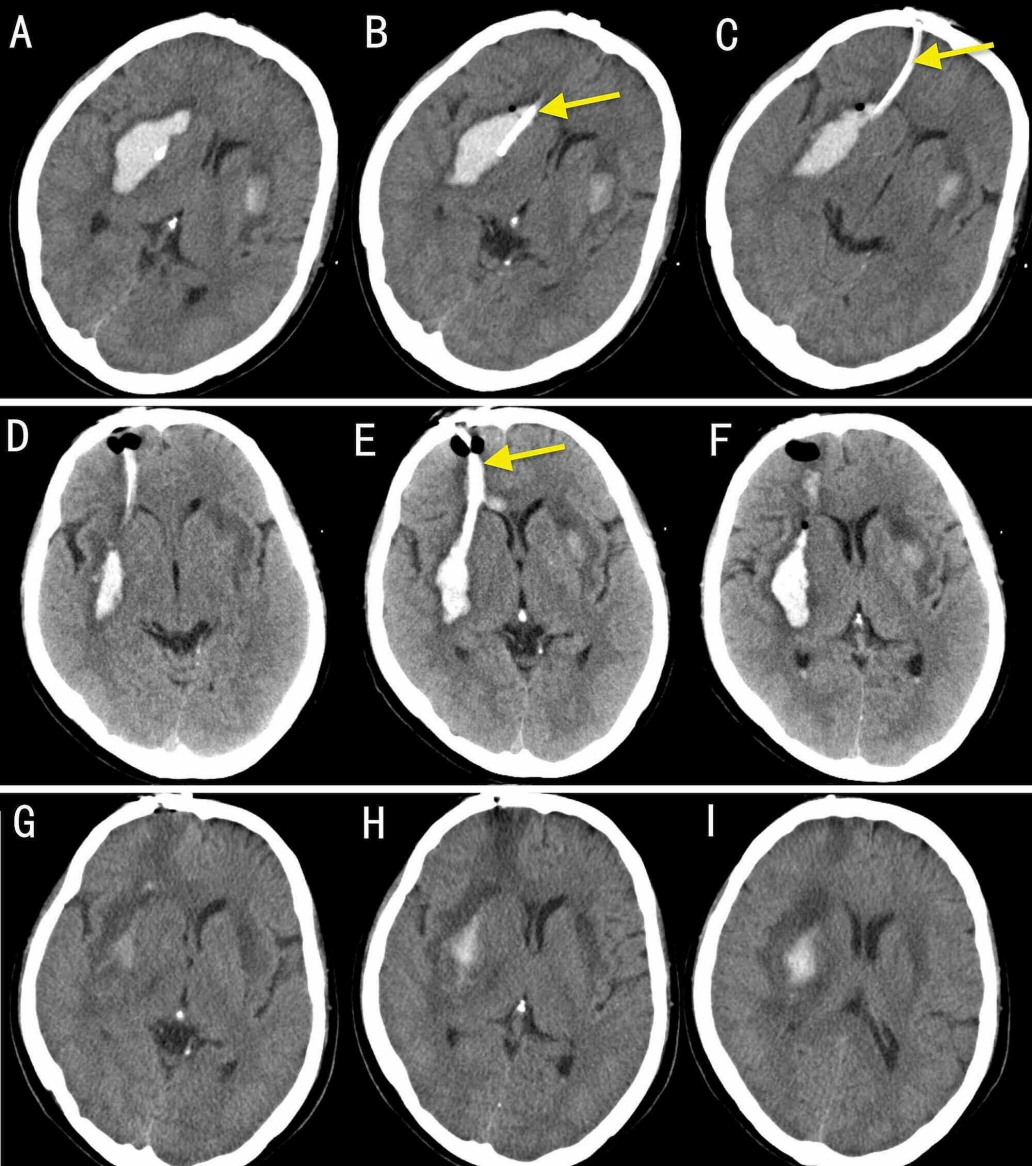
Postoperative axial CT images of the same patient. Postoperative axial CT images of the same patient. Upper row: Postoperative CT image (A, B, C) [Day 2] showing the catheter (yellow arrow) in the cavity. Middle row: D, E, F images [on Day 4] revealing that the residual hematoma had shrunk and showing the location of the catheter (yellow arrow). Lower row: H, I, G images [on Day 13] showing that the right-sided hematoma had almost disappeared and that the catheter had been pulled out.
